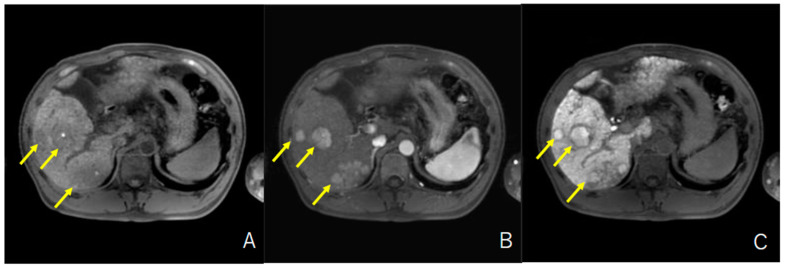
Typical MR images of unresectable HCC with iso-high intensity in the hepatobiliary phase. (A) Plain phase, (B) Arterial phase, (C) Hepatobiliary phase.
